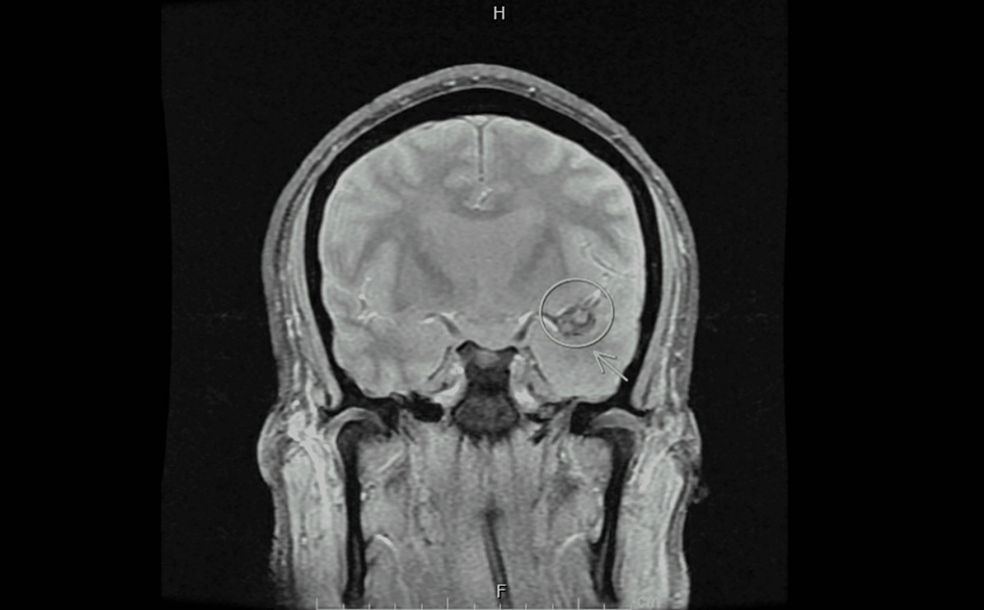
GRE T2-Weighted MRI Brain: Coronal view *Developing hypointense signal abnormality in medial left temporal lobe, suggesting an early hemorrhagic conversion of underlying encephalitis
